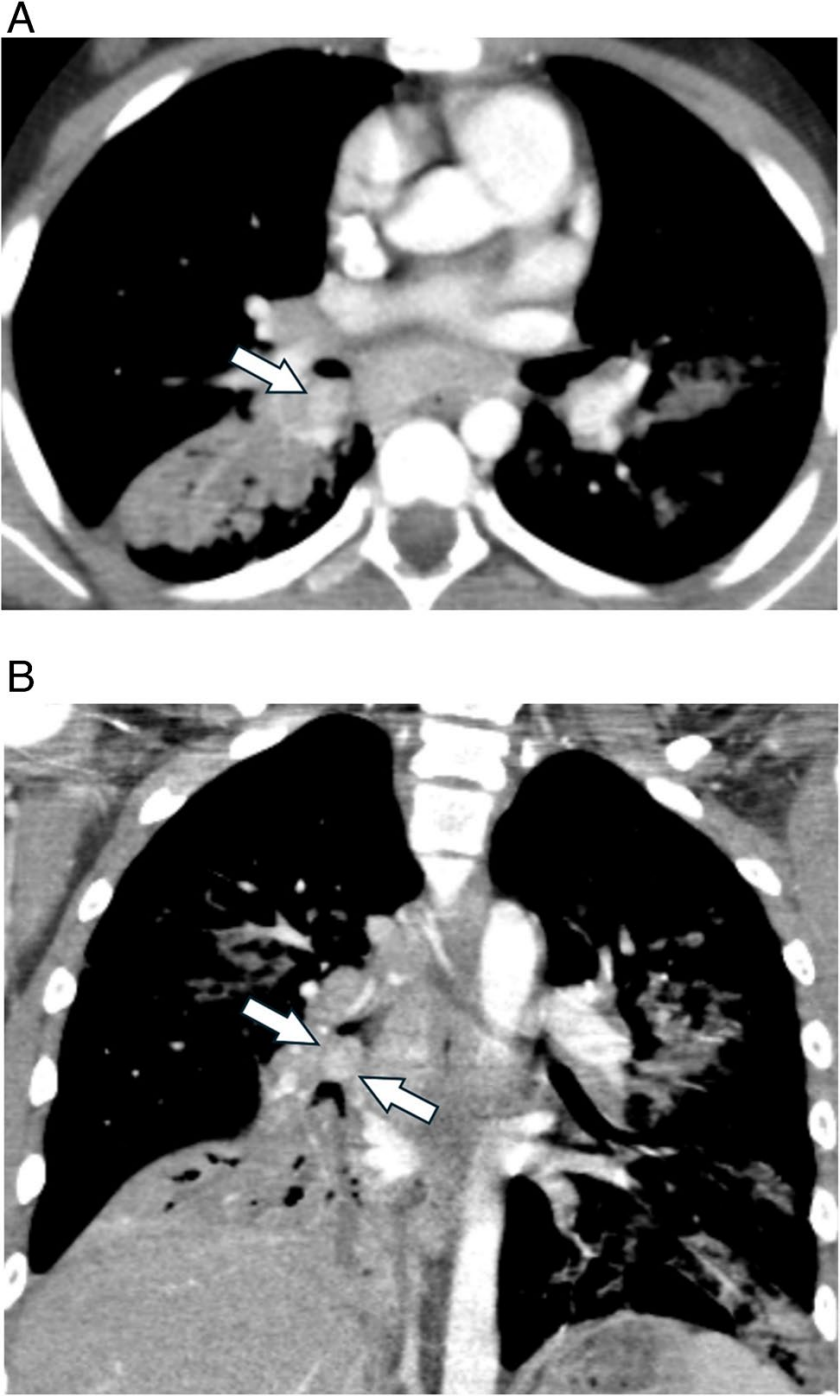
Carcinoid tumor in a 12-year-old female with chronic cough, fevers, and chest pain. A Axial contrast-enhanced chest CT shows an enhancing, endobronchial nodule at the junction between the right lower lobe bronchus and bronchus intermedius (*arrow*). B Coronal CT image shows airways distal to the endobronchial tumor (*arrows*) are mucus filled and dilated, and there is consolidation of the right lower lobe
